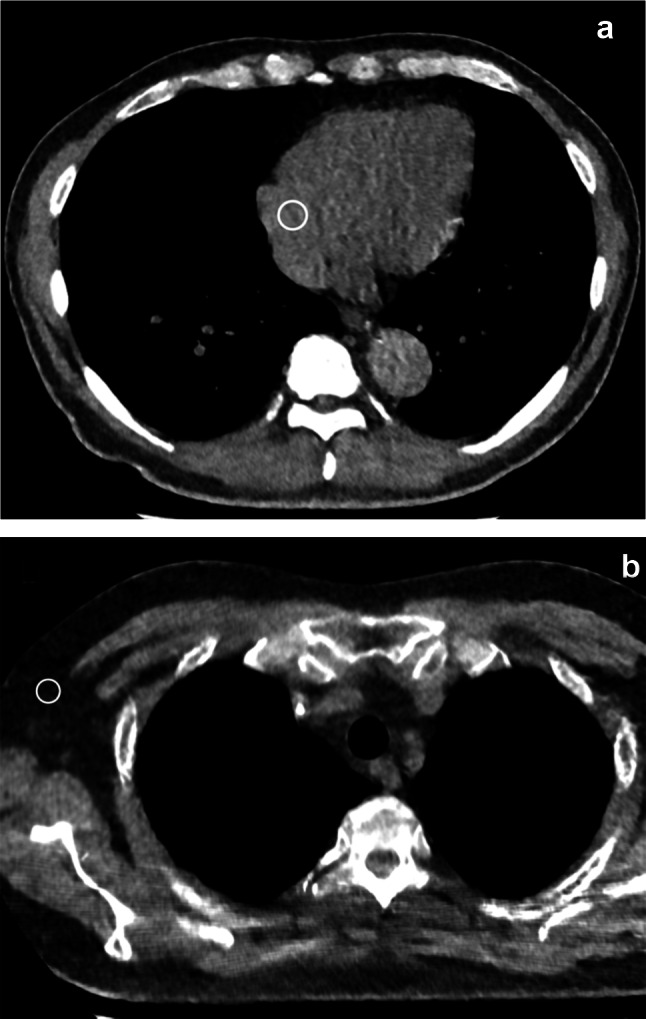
In quantitative image analyses, a circular or ovoid region of interest (white circle) was placed on the right atrium of the heart (a) and subcutaneous fat in the right axilla (b). Subsequently, the mean and standard deviation of CT attenuation within the region of interest on the right atrium and the mean CT attenuation within the region of interest on the subcutaneous fat were recorded
